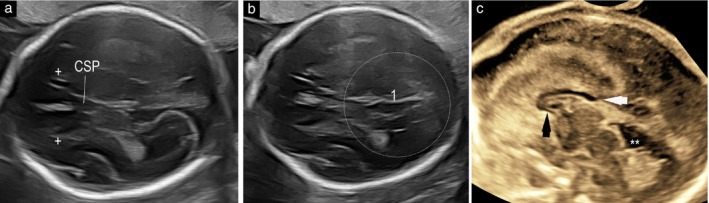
Transabdominal (a,b) and transvaginal (c) ultrasound images in a fetus with partial agenesis of the corpus callosum at 23 weeks' gestation. (a) Image at the level of the anterior complex demonstrating normal triangular appearance of cavum septum pellucidum (CSP) with length‐to‐width ratio of ≥ 1.5. (b) Image at the level of the posterior complex in axial plane demonstrating that the callosal sulcus is absent, parieto‐occipital fissure lacks definition (1) and corpus callosum does not cross the midline. (c) In the midsagittal plane, both the rostrum (black arrowhead) and part of the splenium (white arrowhead) were missing on direct three‐dimensional transvaginal visualization. Arrows indicate where these structures should be observed.
